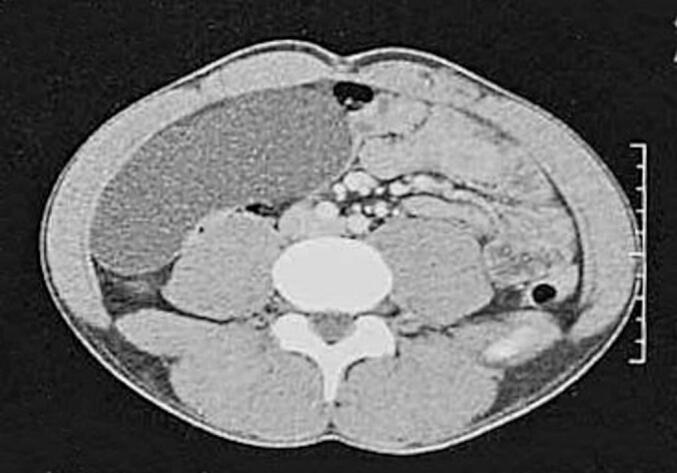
CT scan images of the peritoneal cyst.
